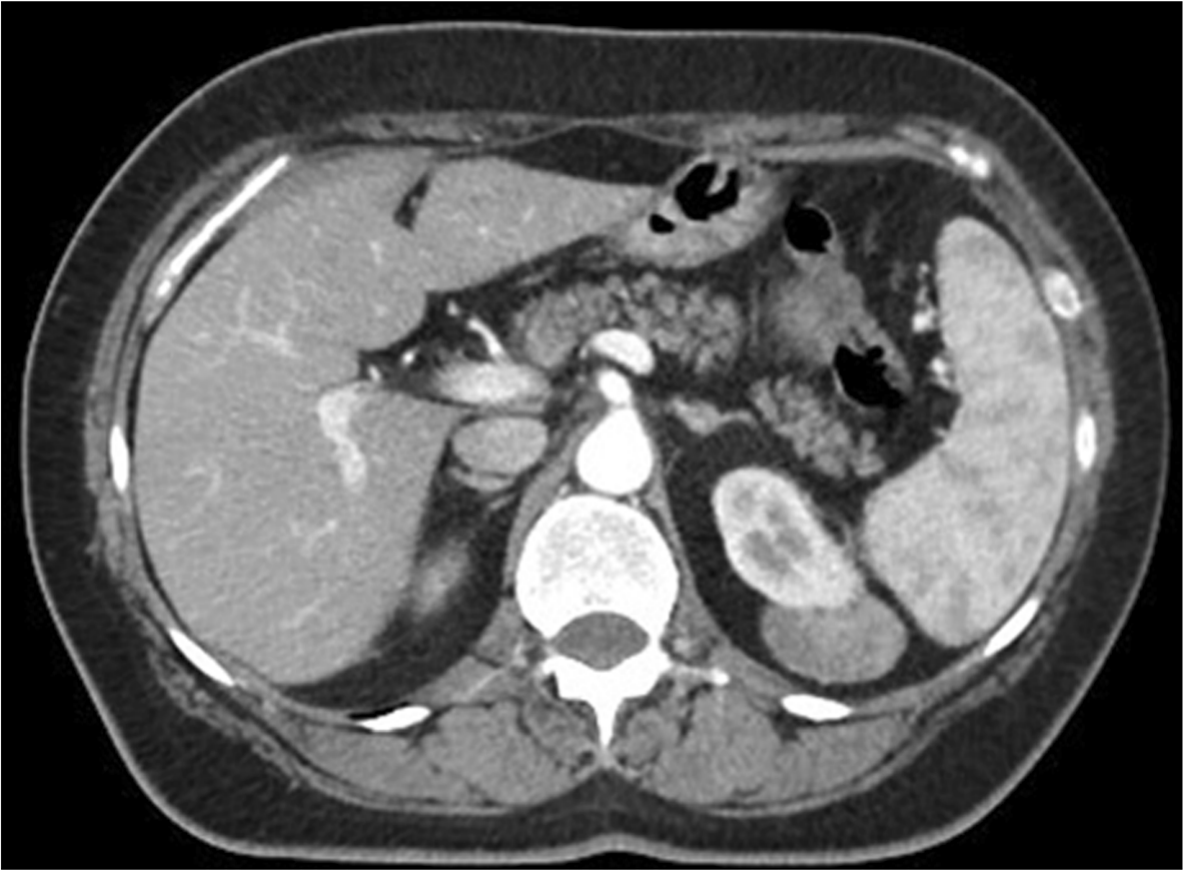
Computed tomography view of renal mass. Computed tomography (CT) scan shows a solid 5 cm mass in the left kidney with exophytic pattern placed in the upper pole of the kidney. The imaging features were consistent with clear RCC
